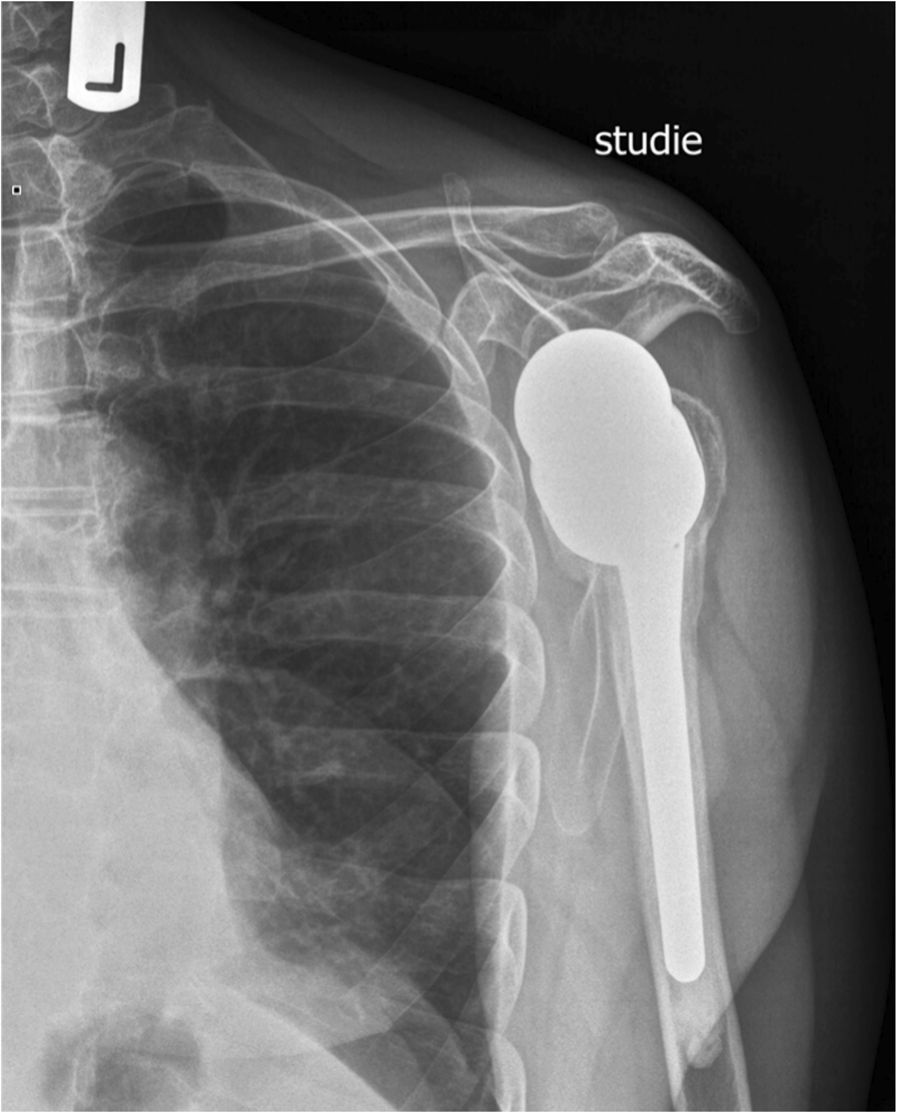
X-ray one year after implantation of reverse shoulder arthroplasty (Y-view).
